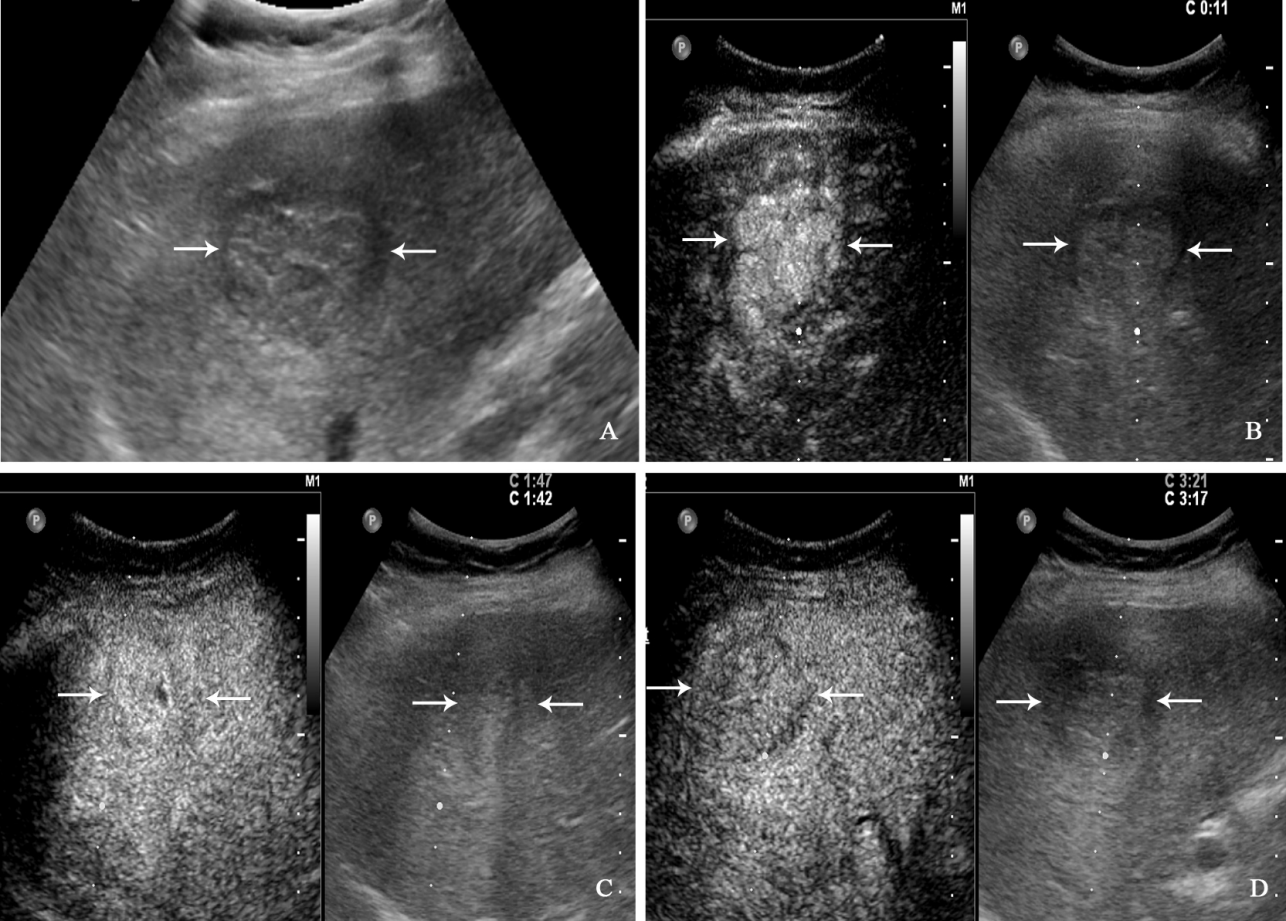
A 71-year-old man with hepatocellular carcinoma. The patient had no chronic hepatitis B and C Conventional ultrasound showed that a hypoechoic tumor with largest diameter of 3.8 centimeters in right liver lobe (A). In the arterial phase of contrast-enhanced ultrasound, the tumor showed homogeneous hyperenhancement (B), and isoenhancement in the portal venous phase (C), finally with slightly washout in the late phase (D).
